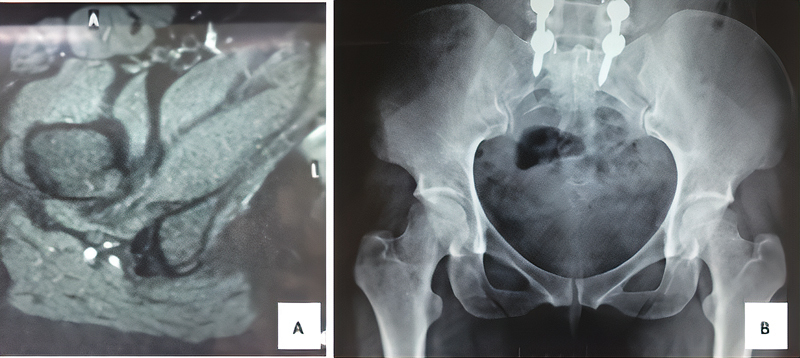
Preoperative nuclear magnetic resonance imaging (
